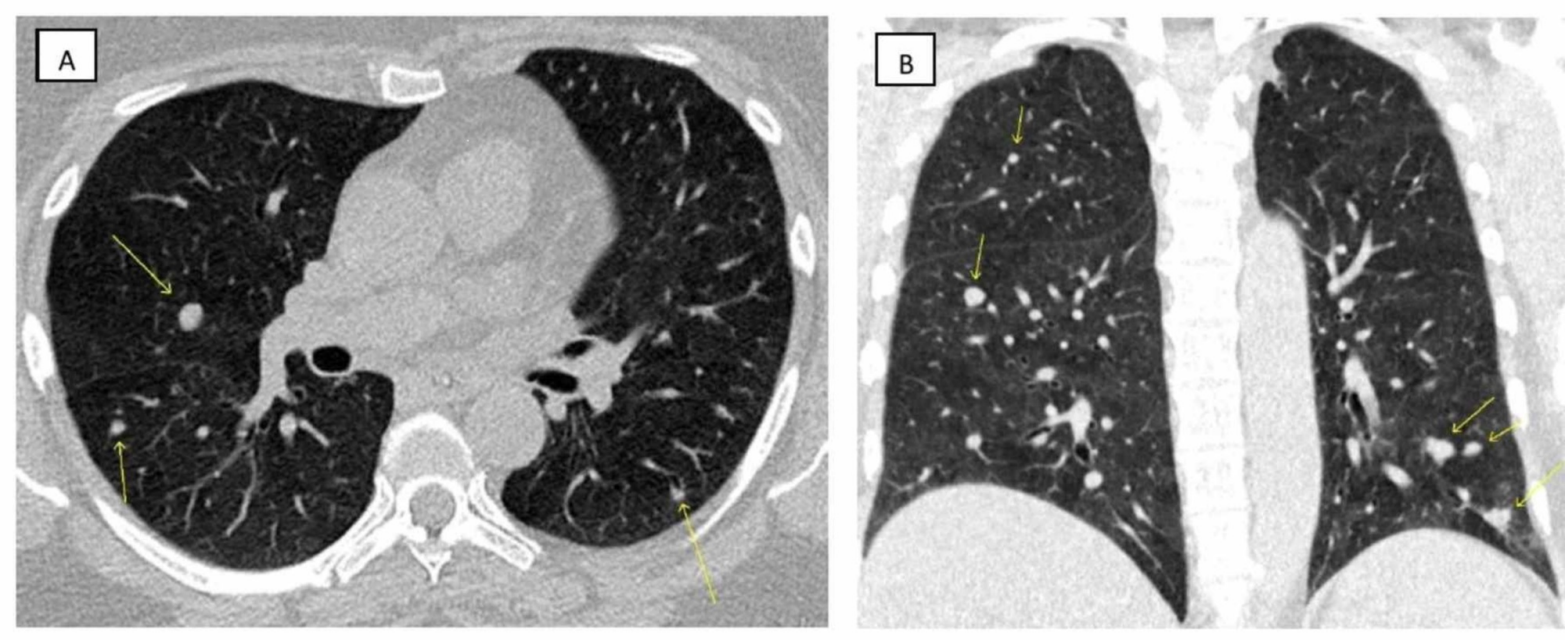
Chest computed tomography A, An axial window of the lung shows a rounded, about 7 mm, prominent nodule in the right lower lobe along with a few other nodules in the right and left lung (arrow); B, A coronal section of the lung shows the presence of multiple lung nodules (arrow)
